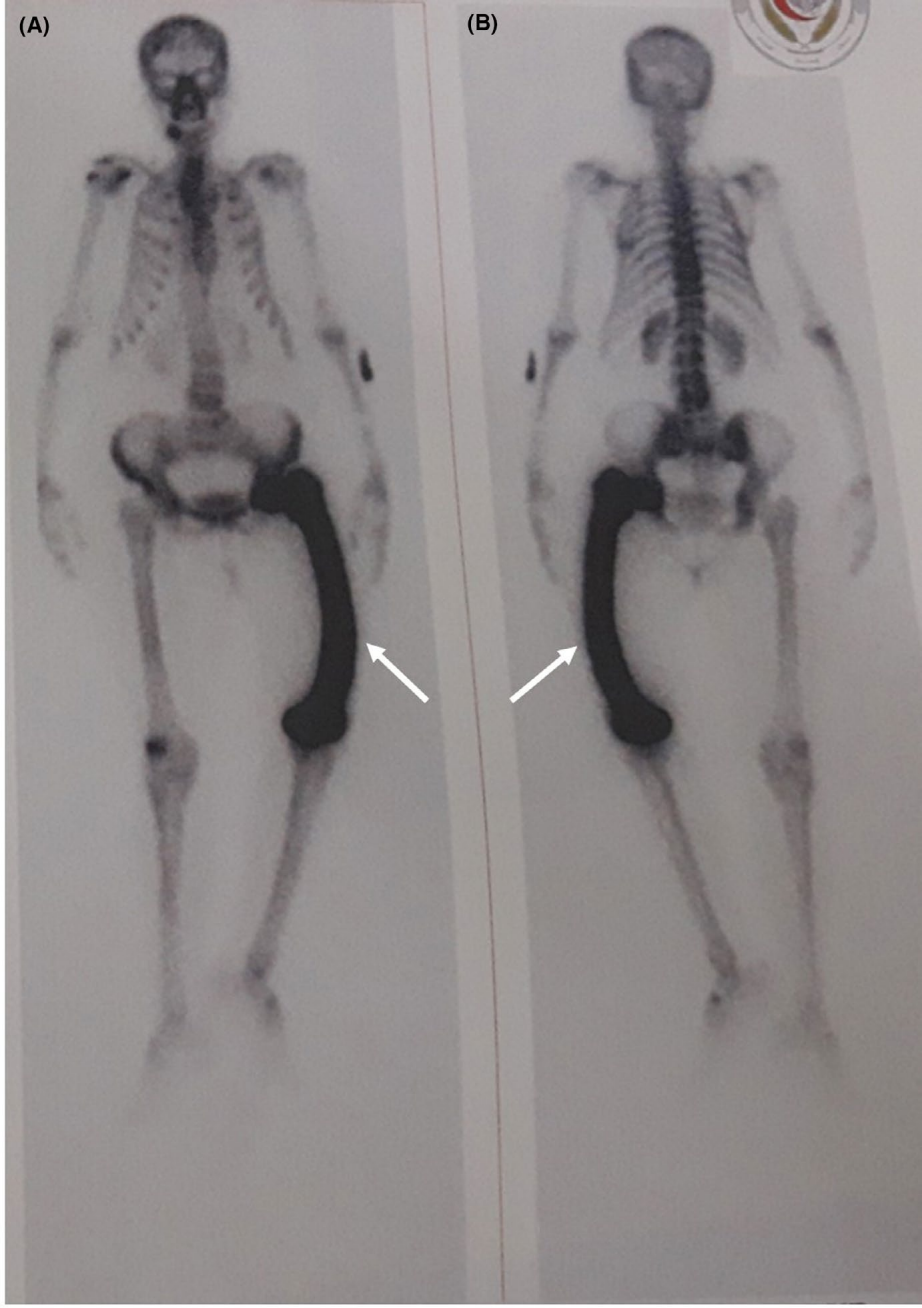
Radionuclide bone scintigraphy revealing diffusely increased homogeneous uptake (arrow) in the left femur with bowing deformity (A: anterior view; B: posterior view)
